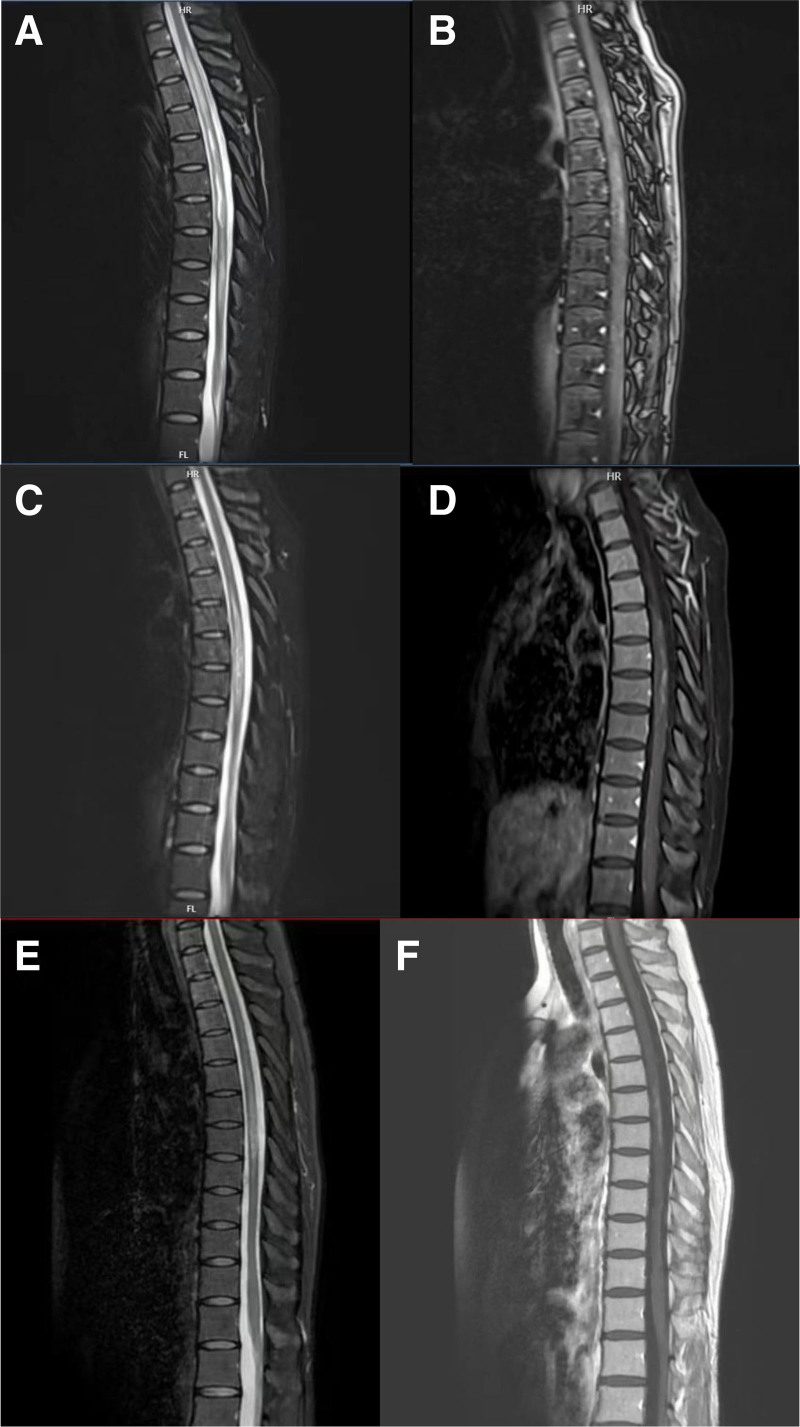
Sagittal magnetic resonance images of the thoracic spine: T2-weighted and T1-weighted imaging with contrast. (A and B) A long demyelinating lesion exhibiting contrast enhancement is seen from T2 to T10, 2 months after her initial presentation. (C and D) Images 4 months later revealing demyelination from T4 to T8. (E and F) Images acquired almost 1 year after diagnosis revealing demyelination from T4 to T7.
